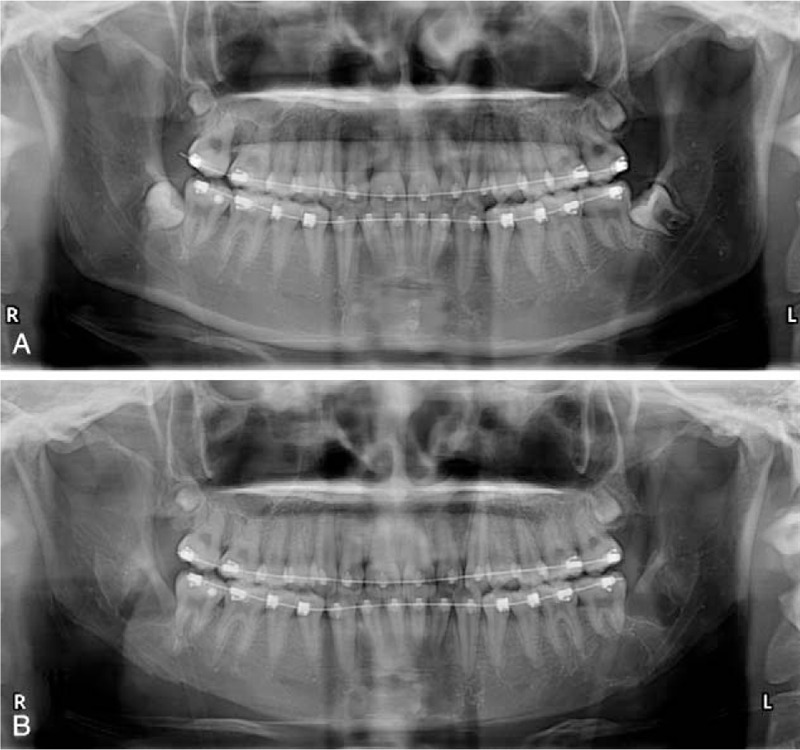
Mandibular panoramic radiographs of patient 1. The relationship among the impacted wisdom teeth, the inferior alveolar neurovascular bundle, and the inferior mandibular margin was clearly shown in the pre-op radiograph (A). Bilateral impacted wisdom teeth were extracted without fracture line in short-term post-op radiograph (B).
